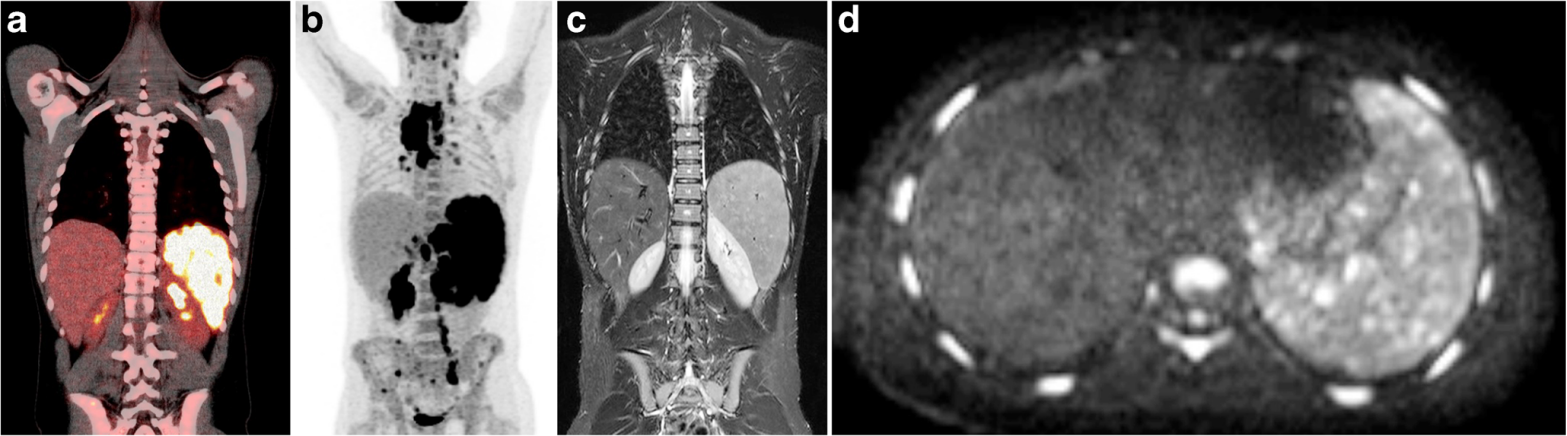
A 13-year-old boy with stage III Hodgkin lymphoma with diffuse splenic disease involvement. a Coronal 18F-fluoro-2-deoxy-D-glucose (FDG) positron emission tomography (PET)/computed tomography (CT) shows diffuse splenic FDG uptake and splenomegaly. b Coronal maximum intensity projection of the FDG-PET shows the extension of disease throughout the body including diffuse splenic infiltration and involvement of various lymph node stations (cervical, mediastinal, para-aortal). c Coronal T2-weighted magnetic resonance image (TR/TE 5,231/65 ms) shows diffuse splenic inhomogeneity and splenomegaly. d Axial diffusion-weighted image (b=800) shows diffuse inhomogeneity of the spleen
